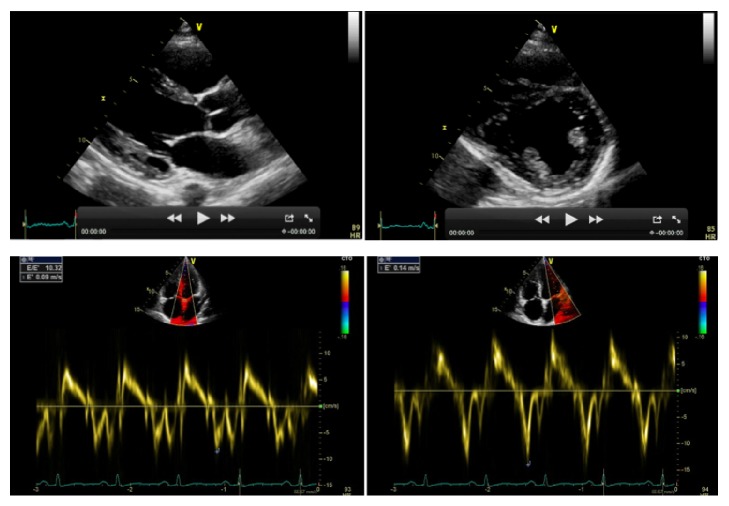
Transthoracic echocardiography.* Almost complete resolution of the pericardial disease after 5 weeks of therapy with glucocorticoids.
